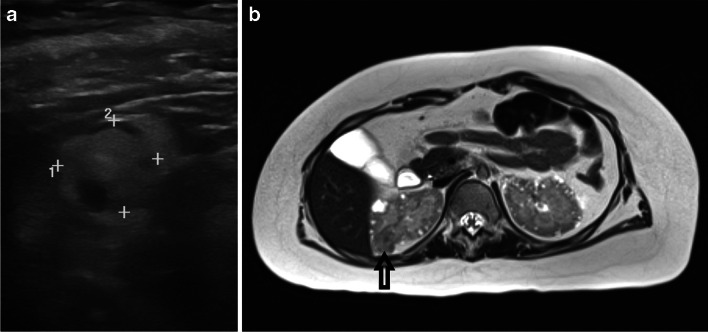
a, b Comparison of kidney lesion visualisation in TSC between US and MRI. Arrow on T2-weighted MRI image in right panel shows the lesion which is represented on left US panel, lesion measures 18 mm × 17 mm in Fig. 1a
